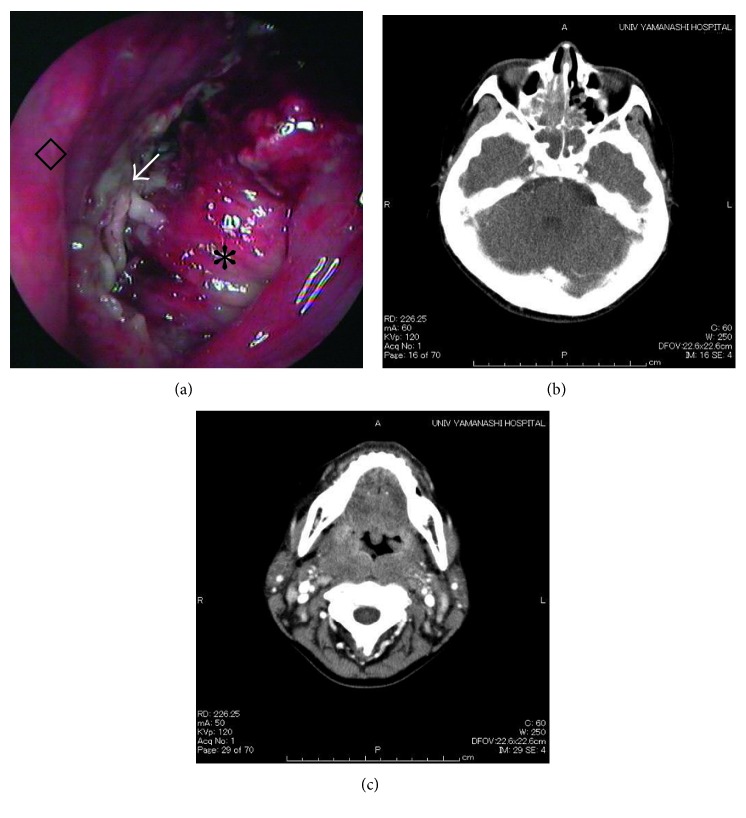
Scope image and computed tomography (CT) findings. (a) Scope image of the left nasal cavity. White granulation tissue is observed (arrow). ^∗^: inferior turbinate and ◇: nasal septum. (b) Axial CT with contrast demonstrating soft tissue lesions or effusions in the ethmoid and sphenoid sinuses. (c) Axial CT with contrast demonstrating mucosal and tissue swelling in the pharynx.
